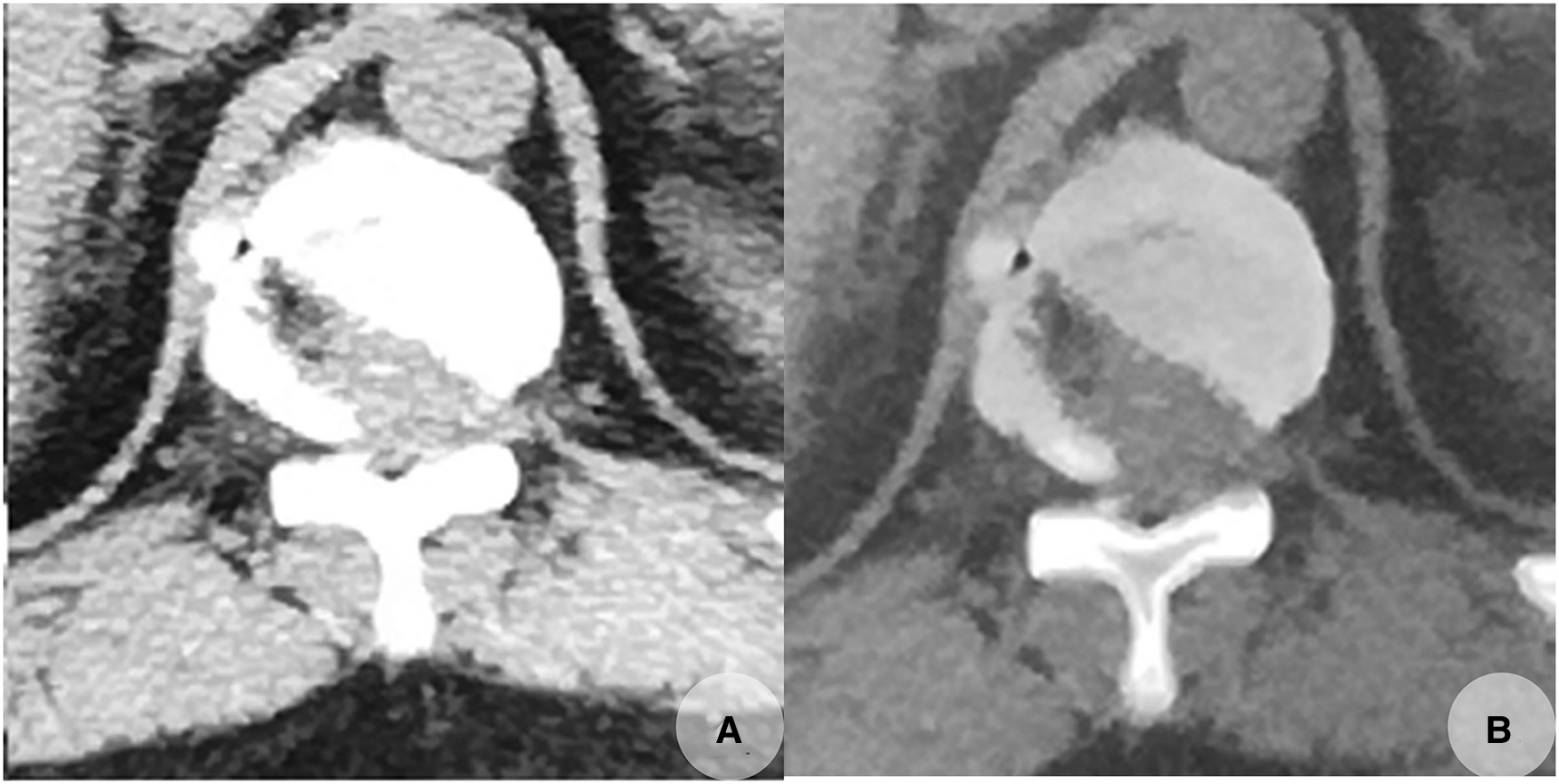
Postoperative CT scans taken on November 23, 2019, revealed that the calcified herniated disc was completely removed with some normal bone excessively removed (A,B). (A,B) Transverse CT images of T11/12.
